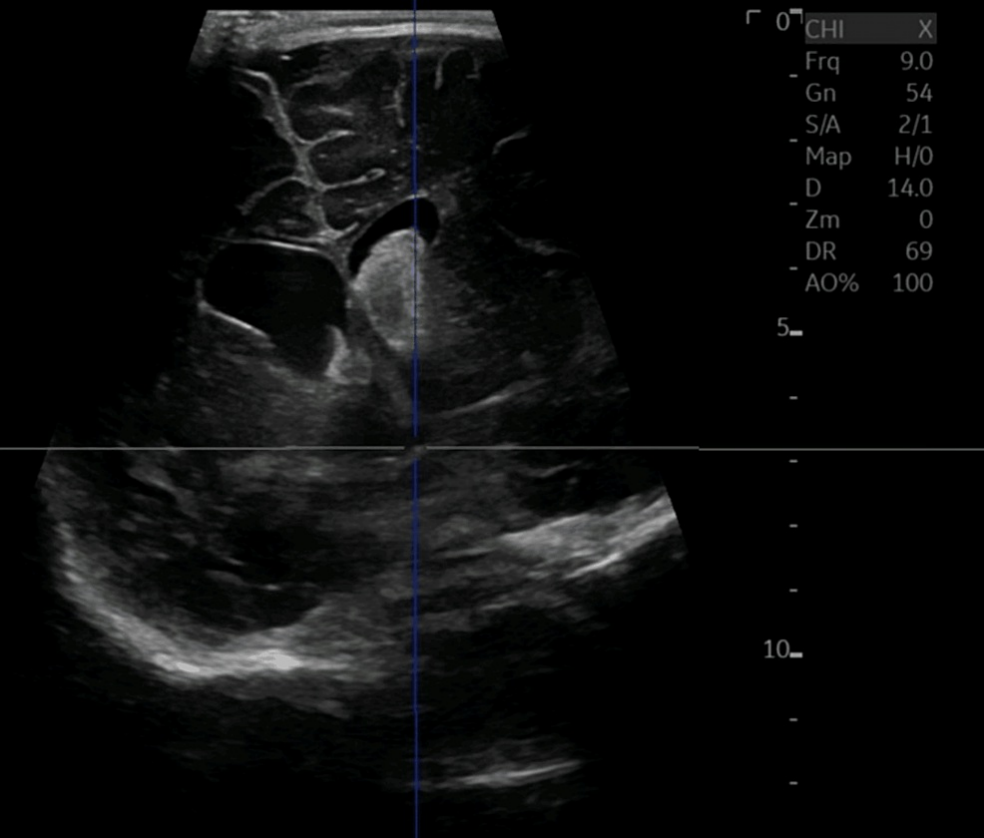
Ultrasound of the head depicting intraventricular hemorrhage.
